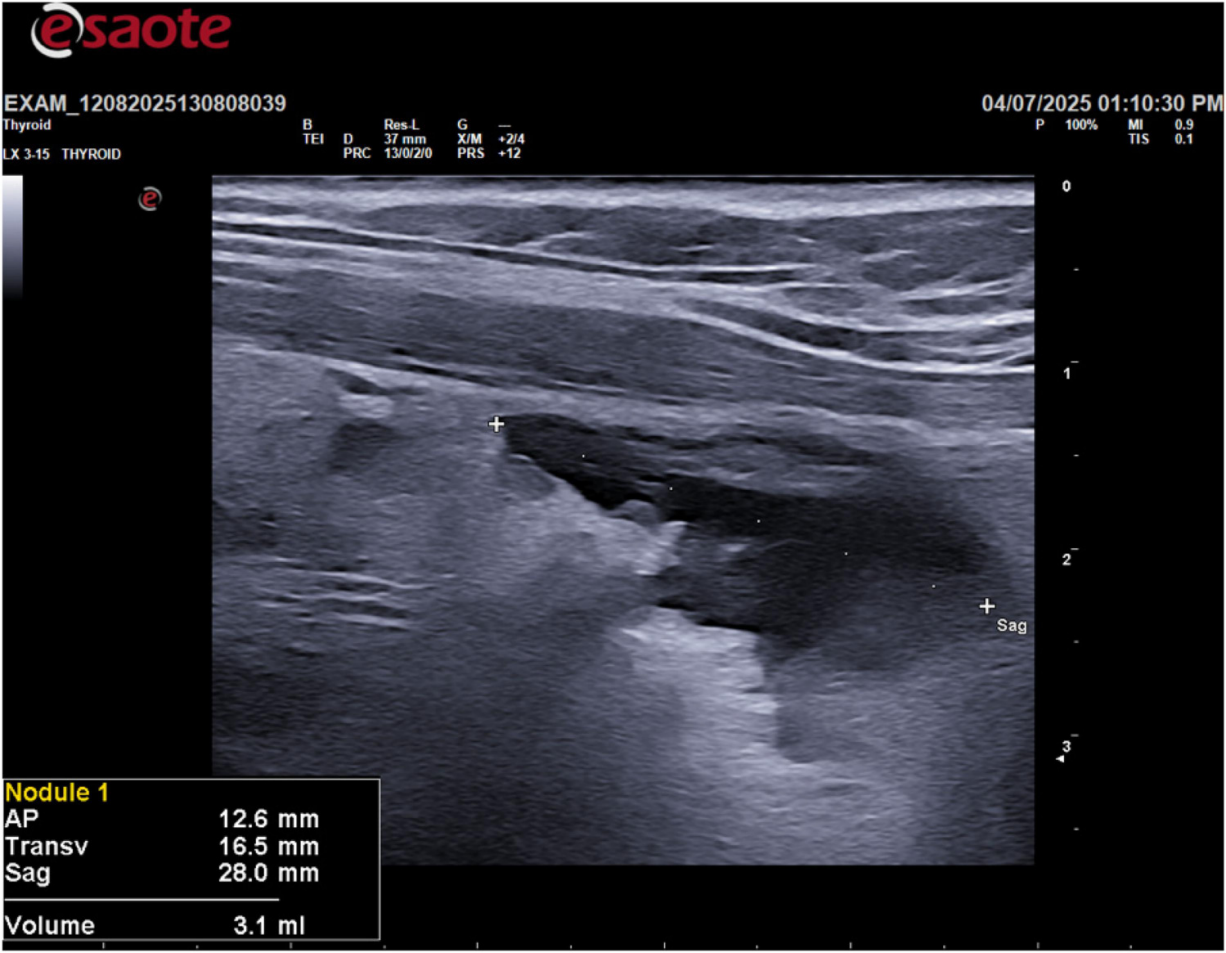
Post fine-needle aspiration (FNA) ultrasound image.
